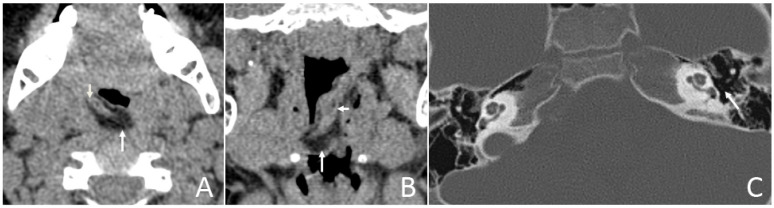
CT of a 2-year and 11-month boy presenting with snoring and mouth breathing for 3 months and hearing impairment found during physical examination. (A,B) Axial and coronal CT displayed in the soft-tissue window show the fat density mass (long arrow) originating from the back side of the left soft palate with a soft tissue density core (short arrow). (C) Axial CT, displayed in the bone window, shows the absence of the left stapes head and part of the stapes arch (arrow).
